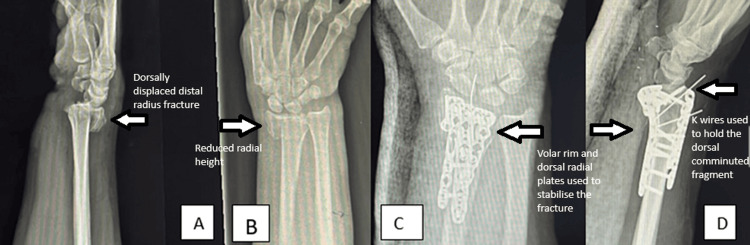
Plain radiograph of the wrist joint A, B: Plain radiograph post trauma showing AO 2R3C2 fracture; C, D: Postoperative radiograph
